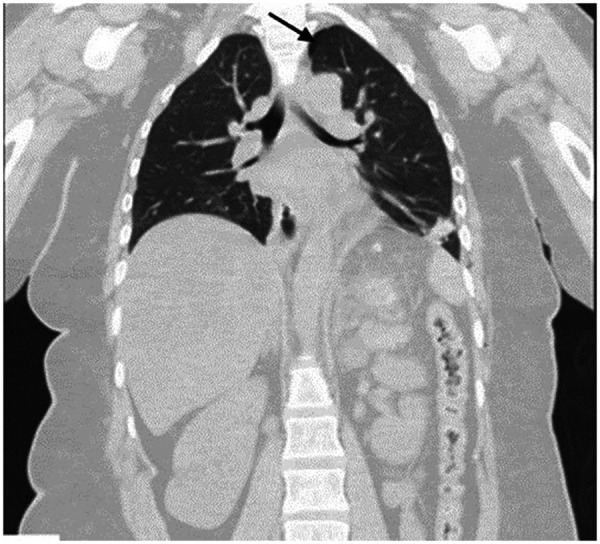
Contrast-enhanced CT chest (coronal view). Black arrow indicates mild left-sided pneumothorax at the upper lobe, with associated bilateral pleural effusions.
